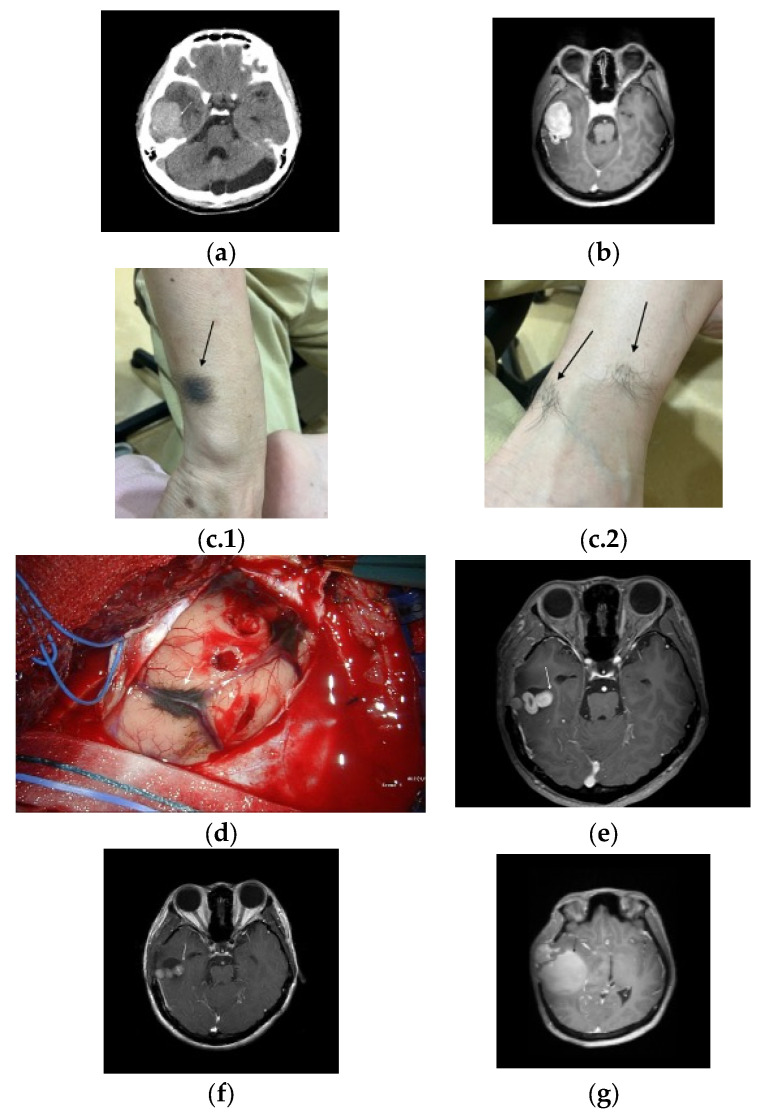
(a) Brain computed tomography with contrast shows a mass (arrow) in the right temporal lobe accompanied by perifocal edema, along with an incidental arachnoid cyst identified in the cerebellar region. (b) Brain magnetic resonance imaging with contrast shows a well-defined, intensely and homogeneously enhanced mass lesion (arrow) in the right temporal lobe. (c.1,c.2) Multiple pigmented skin lesions (arrows).(d) Intraoperative photograph shows a hypervascular black-colored tumor (arrow) and marked adhesions in the temporal lobe and petrosal vein. (e) MRI with contrast (two-months postoperative follow-up) showed small residual tumor (arrow). (f) MRI with contrast (three months post-gamma knife radiosurgery) shows residual tumor (arrow). (g) MRI with contrast showed tumor recurrence with cystic changes (arrow).
